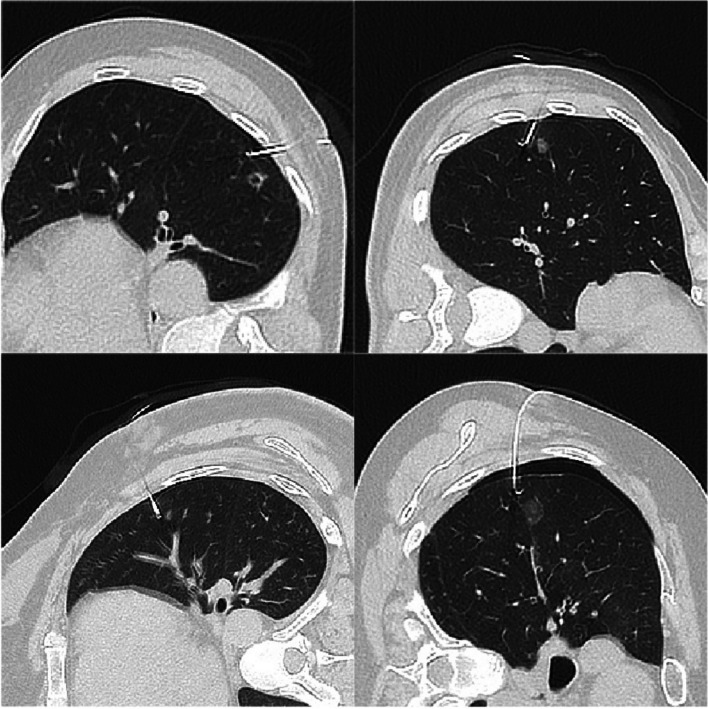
CT scanning was performed after the localization to confirm the accuracy
